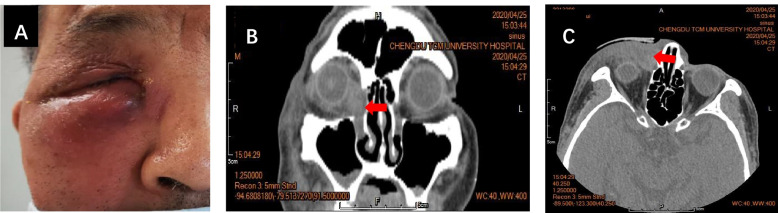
The patient’s eye appearance and results of the CT scan of the sinuses. A The patient’s condition at admission. Redness and swelling in the right lacrimal sac area are observably visible and without fluctuation. B The coronal CT image. A comparison between the left and right lacrimal sac areas; swelling of the soft tissue in the orbit and lacrimal sac areas of the right eye are clearly visible (as shown by the red arrow). C The horizontal CT image shows thickening of the soft tissue opacity in the right lacrimal sac and face area (as shown by the red arrow)
